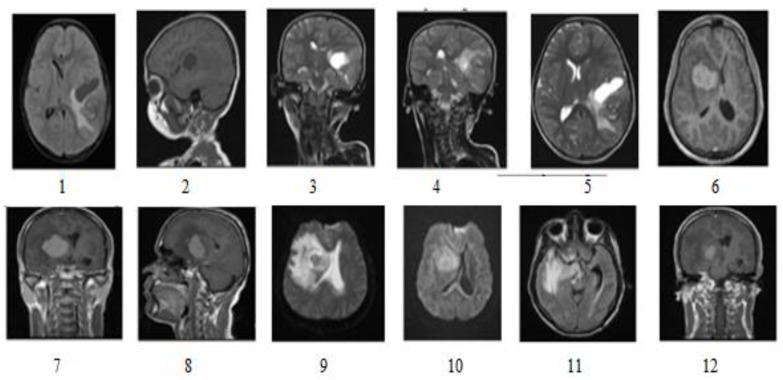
Dataset Includes different types of image sequences 1–12 (T1, T2, and FLAIR) [22].
